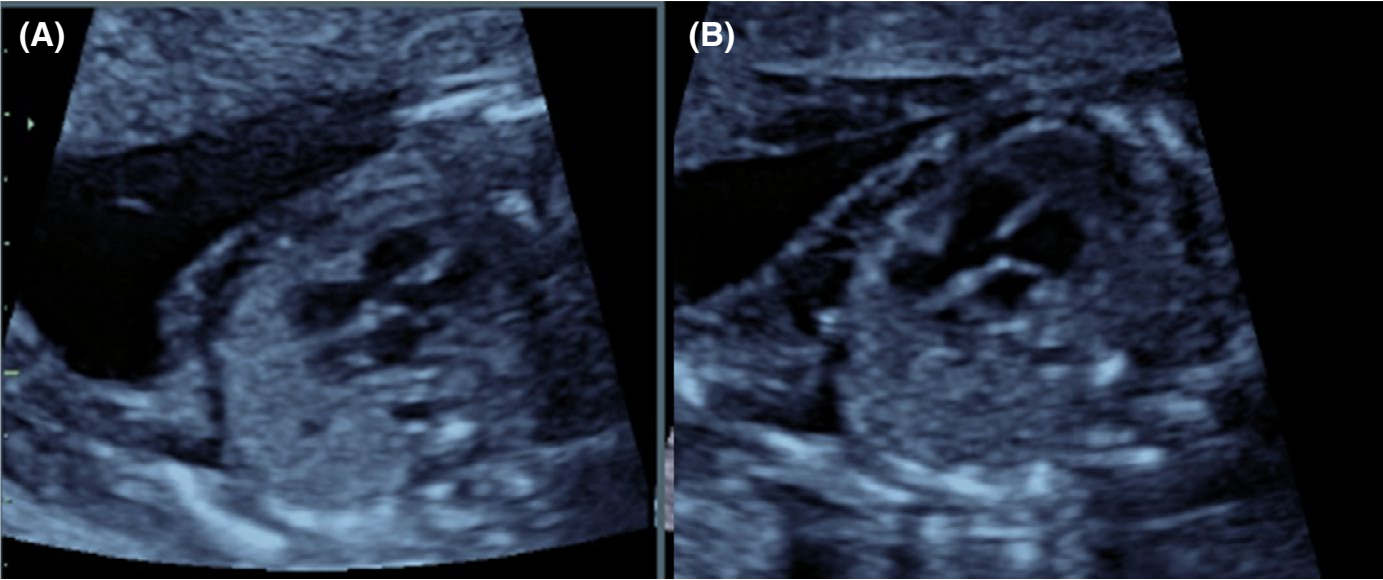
Ultrasound examination at 18 WG. (A) Suspected interventricular communication. (B) Common arterial trunk
